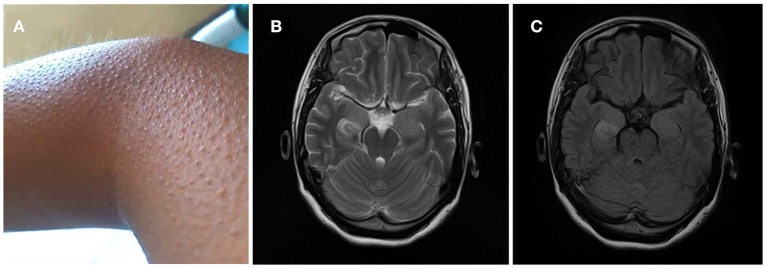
(A) Frequent piloerection involved the patient's limbs, lasted from a few seconds to >2 min, and occurred several times throughout the day. (B,C) MRI T2-weighted and flair images showed signal change on right hippocampus.
